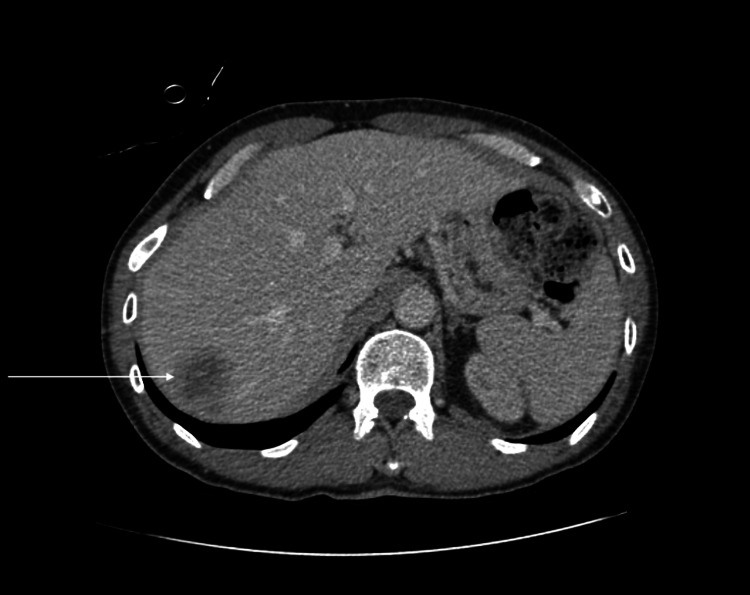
CT scan of the abdomen showing indeterminate complex hypodense lesion at the posterior right hepatic lobe measuring 3.6 x 3.6 cm (white arrow)
